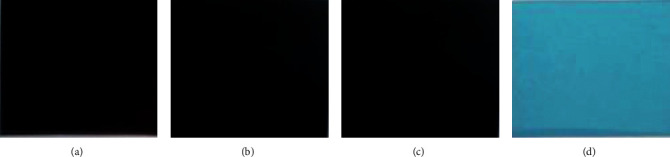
Removal of gelatin and recovery of silver from X-ray films from crude bromelain. Used X-ray films (a) and (b) were collected from Dessie Referral Hospital and (c) and (d) were collected from other health centers that have not used digital X-ray processing machines. Used X-ray films, (a) and (c), were control treated with phosphate buffer only, whereas (b) and (d) were enzyme (20 ml = 144 U) treated films.
